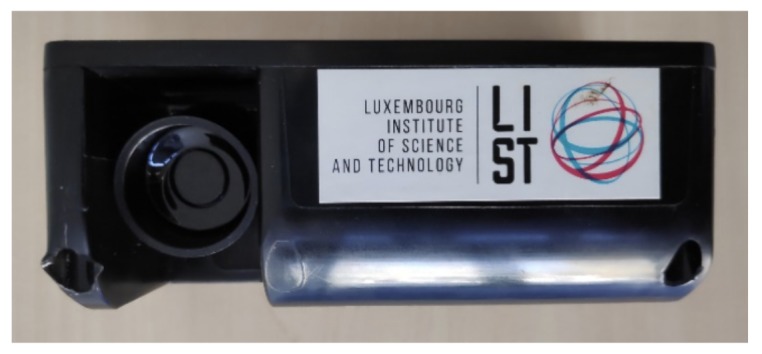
Brighterbins ultrasonic sensor (L=130mm, W=70mm, H=53mm).
